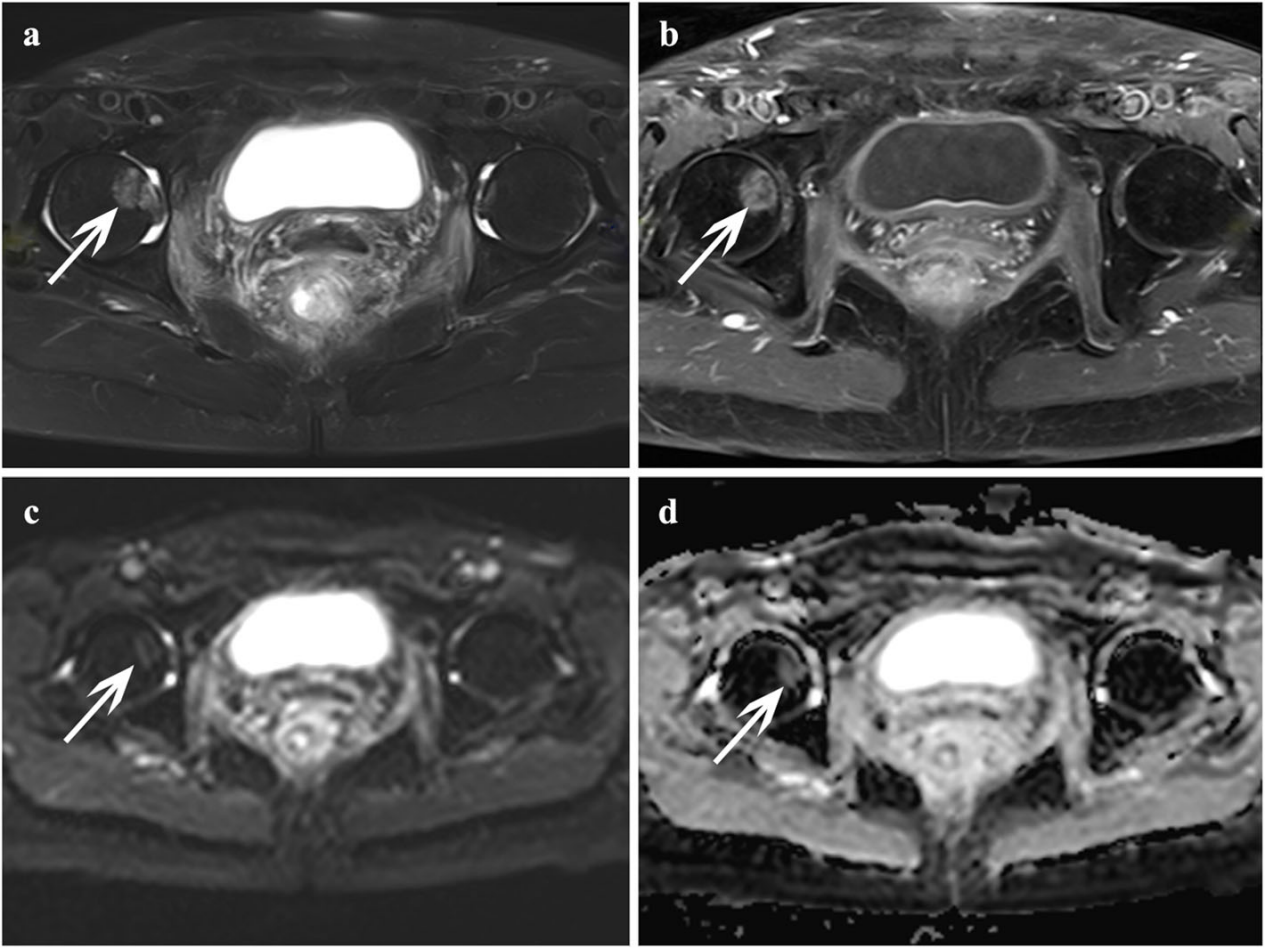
A 66-year-old female with cervical cancer after radiotherapy that was diagnosed with occult IFs (no fracture line detected on all images) of the right femoral head. a Axial FS T2-weighted image showed heterogeneous hyperintensity in the right femoral head (white arrow); b Contrast-enhanced T1-weighted image revealed marked and heterogeneous enhancement change. c The lesion showed equisignal or slightly high signal intensity on DWI, and hyperintensity on ADC map
